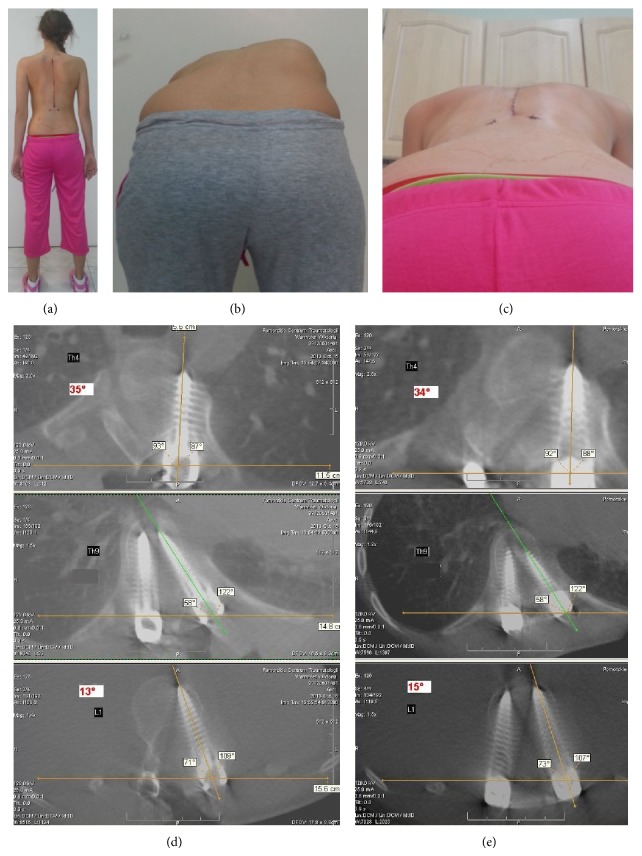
“Patient 13:” (a), (b), and (c) good clinical outcome. (d) Precorrectional CT scan of the same patient: upper window (UIS), middle (AS), lower window (LIS), AS  ( °) − UIS  ( °) = 35° (bigger window in upper section), AS  ( °) − LIS  ( °) = 13° (bigger window in the lower section). (e) Postcorrectional CT scan worsening of AVR after correction, AS  ( °) − UIS  ( °) = 34°, AS  ( °) − LIS  ( °) = 15°, AVR  precorrectional  ( °) = A&D  ( °) = 26°, AVD = (34° ± 15°)/2 − (35° ± 13°)/2 = 0,5°, AVR postcorrectional (°) = 26° + 0,5° = 26,5°. Poor ICT outcome in contrast with Perdriolle AVR.
